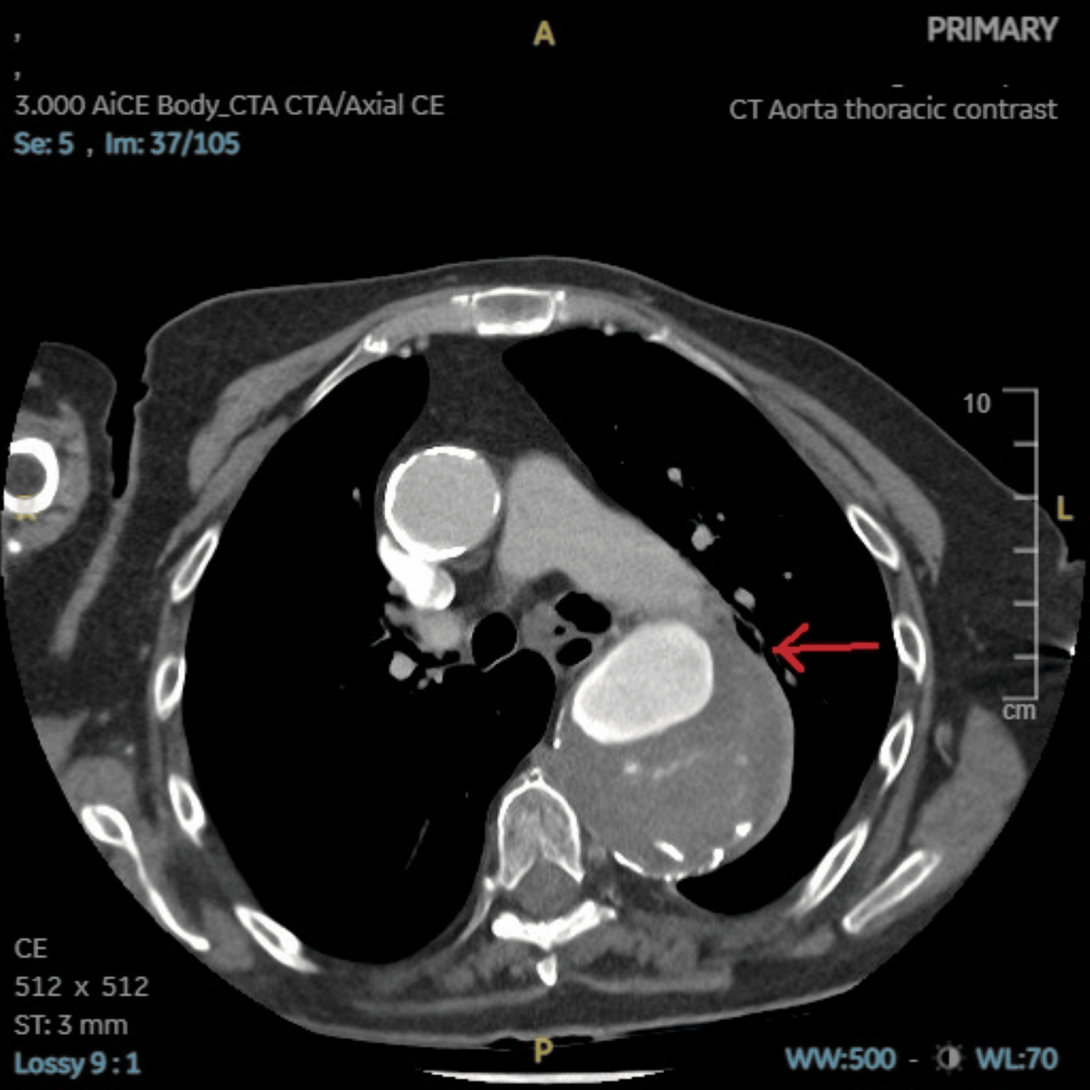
CT aorta with contrast axial view showing a large ruptured aneurysm as highlighted by the red arrow.
